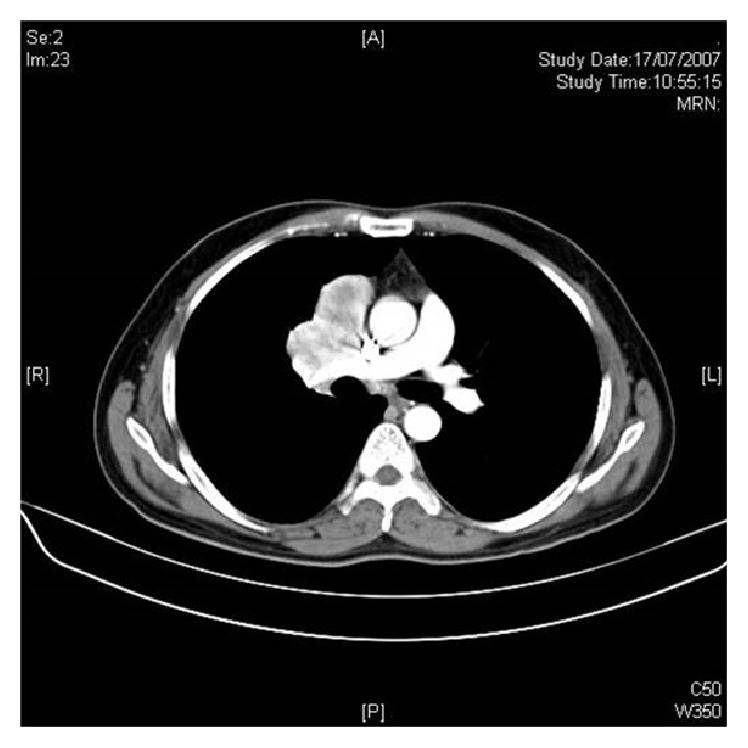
CT thorax showing densely enhancing anterior and middle mediastinal mass.
